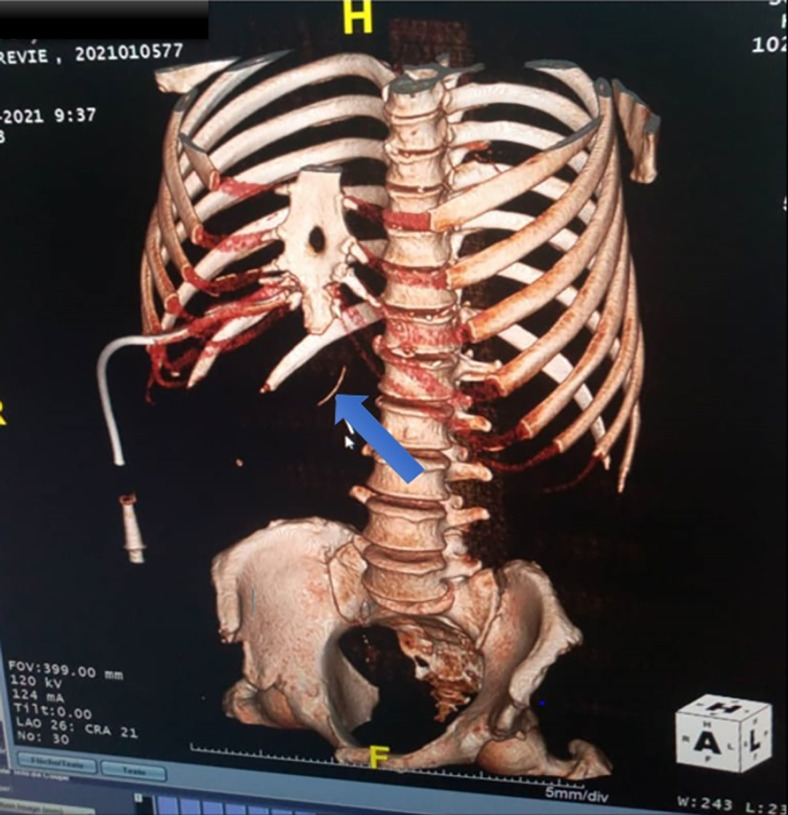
Tomodensitométrie abdominale en reconstruction 3D montrant un corps étranger (flèche bleue) Abdominal computed tomography in 3D reconstruction showing the foreign body (blue arrow)
